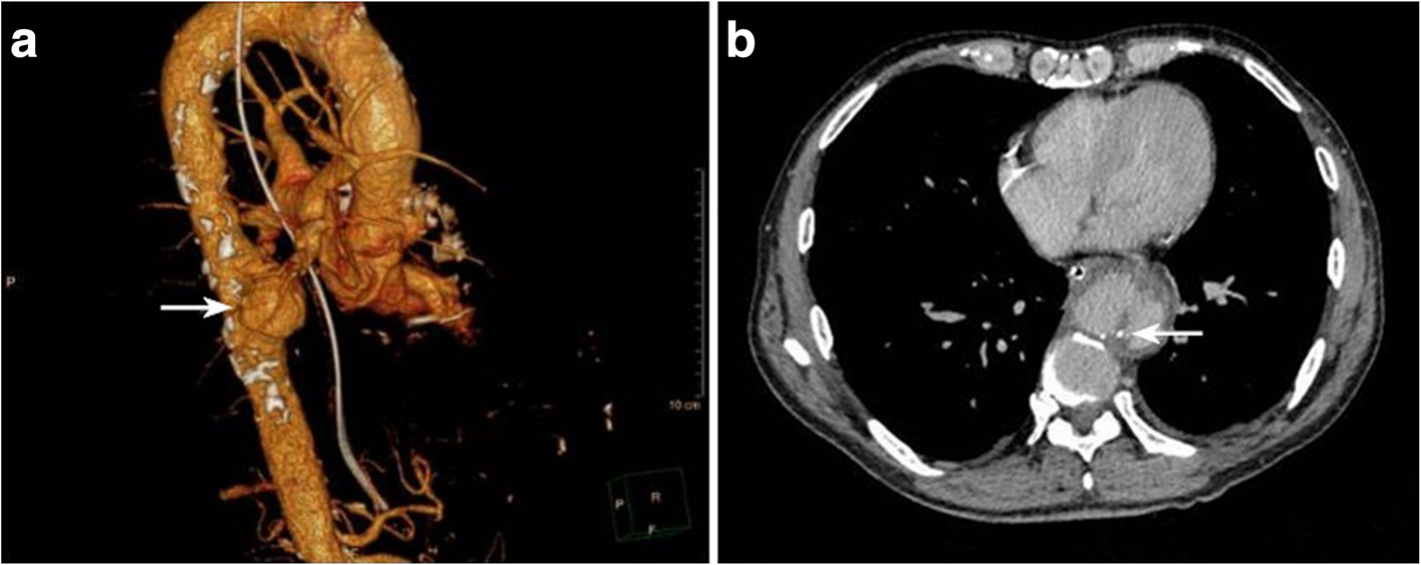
Computed tomography angiography (CTA): a. The pseudoaneurysm was ound in the descending segment of the thoracic aorta, with a maximal diameter of 54 mm (*arrow*). b. Arteriosclerotic plaques were observed in the artery wall (*arrow*)
